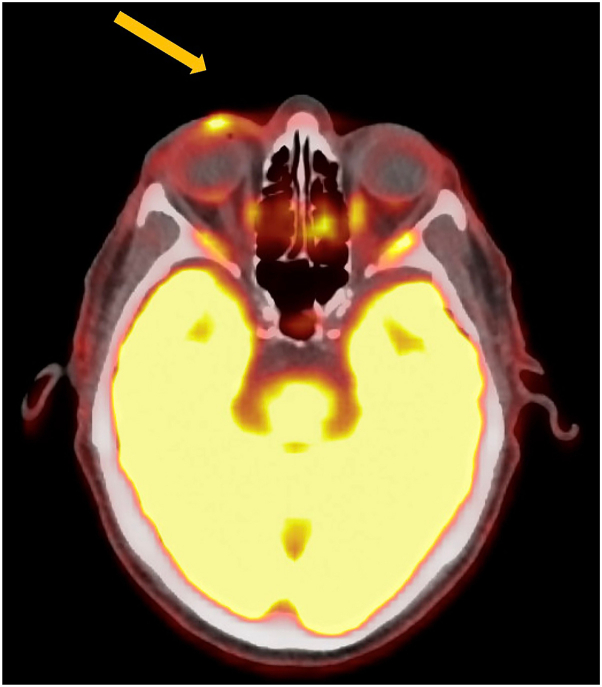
PET/CT 16 days after SFRT. The mass was no longer palpable and was markedly reduced in size, although a focus of glucose uptake (*arrow*) was present after SFRT. Eight days later, excision of this area showed no viable tumor cells. *PET/CT*, Positron emission tomography/computed tomography; *SFRT*, single-fraction radiotherapy.
